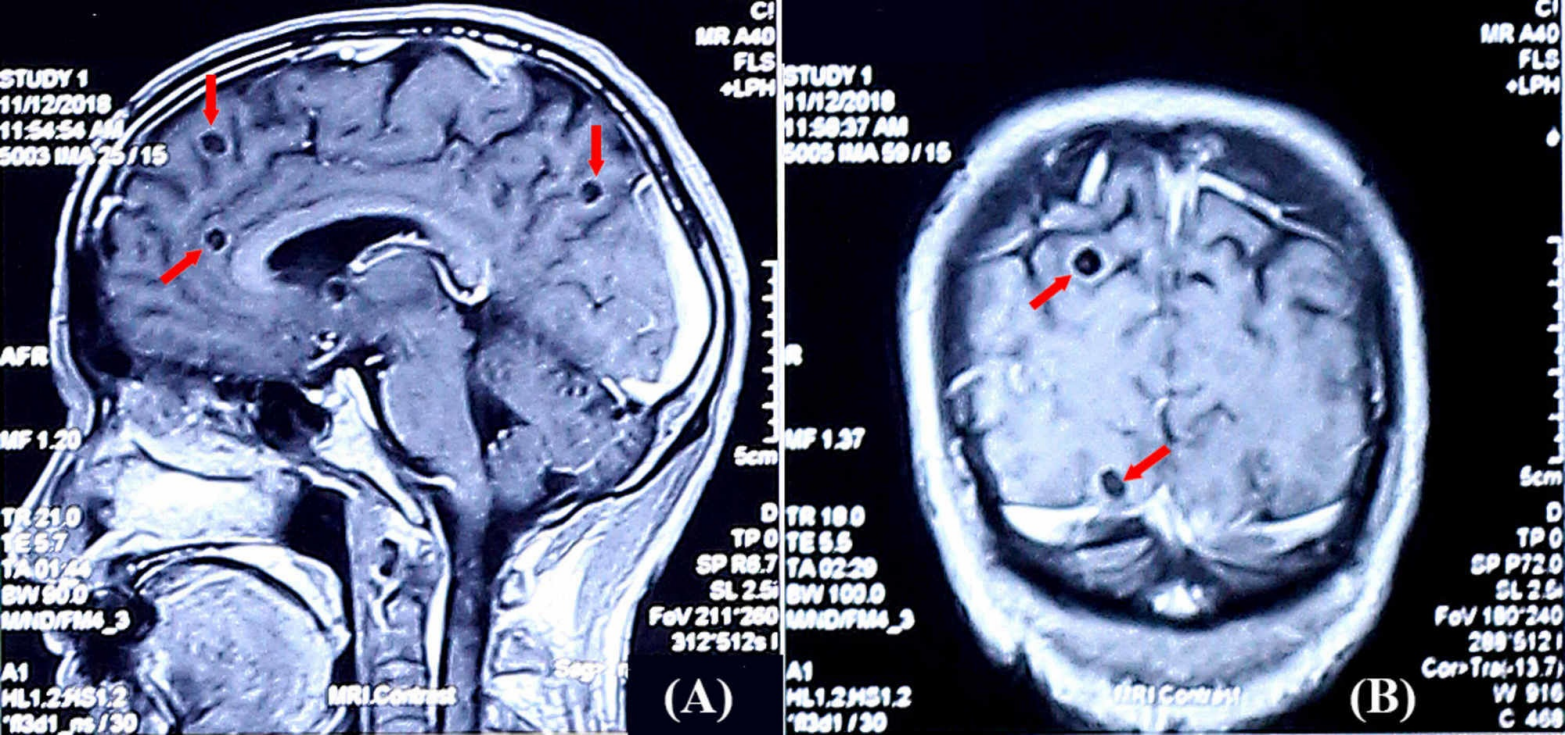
MRI findings of case 2 The sagittal T1 weighted image after contrast showed well-defined ring-enhancing cystic lesions (5-10 mm) in the cerebral hemisphere (A). The coronal T1 weighted image after contrast showed well-defined ring-enhancing cystic lesions (7-9 mm) in the cerebral hemisphere. Scolexes were demonstrated inside the lesions (B) MRI: magnetic resonance imaging
